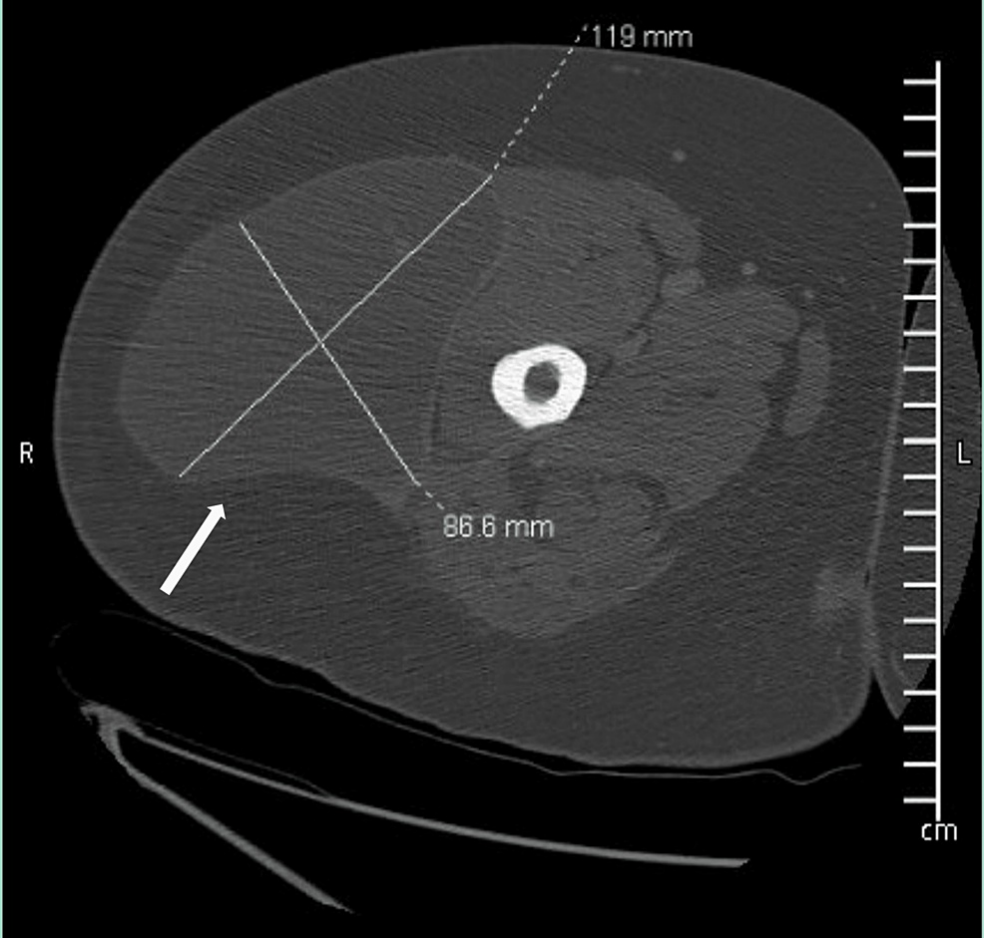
A CT of the right femur with contrast shows rim-enhancing fluid collection measuring 119 x 86.6 mm along the lateral aspect of the thigh.
